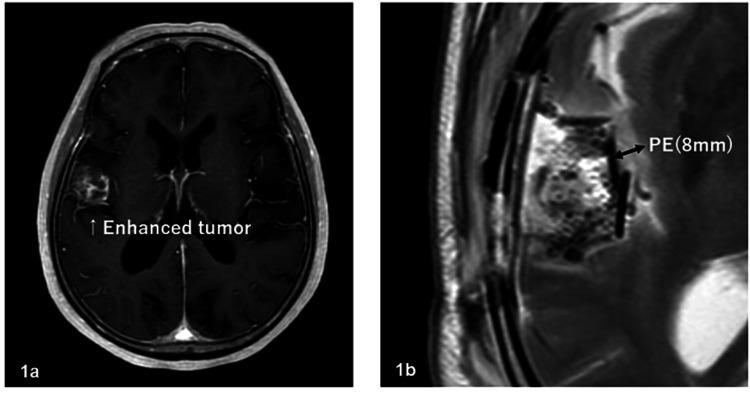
Supplementary MRI images – 1 1a: Gd-enhanced tumor before surgery; 1b: 8-mm PE after surgery MRI: magnetic resonance imaging; PE: preoperative edema
